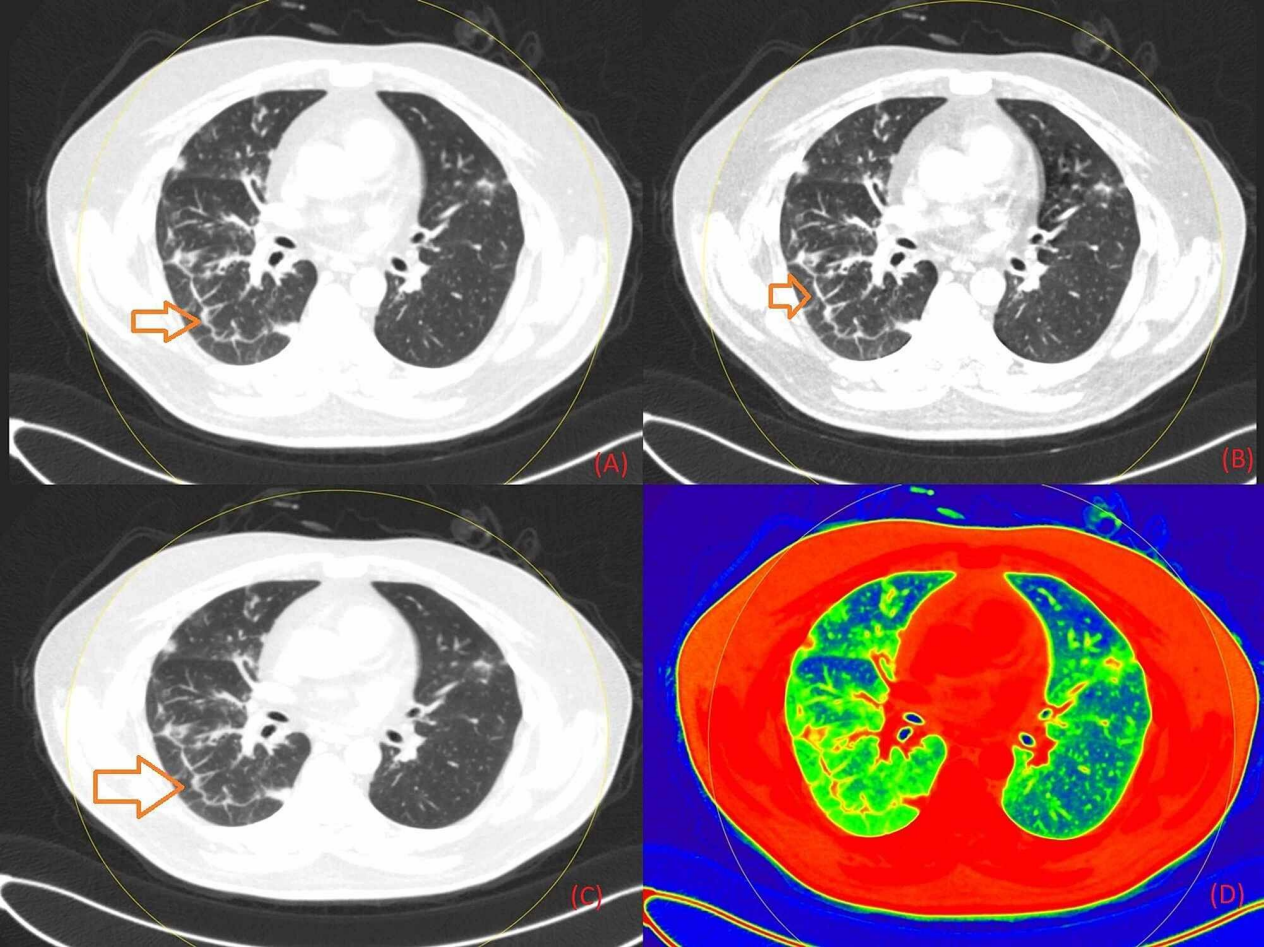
Non-contrast chest DECT axial images representing fibrosis interspersed with GGO in the lower lobe of the right lung field (marked with red arrows) in grayscale (Figure 1A), on 40 keV energy (Figure 1B), on 190 keV energy (Figure 1C), and on color-coded presentation on basis of atomic number (Figure 1D) DECT: Dual-Energy Computed Tomography; GGO: Ground-Glass Opacity
